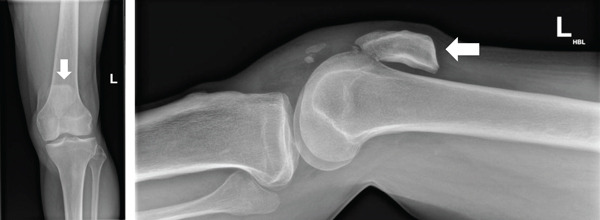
Left knee radiograph demonstrating superior displacement of the patella (arrow), consistent with patella alta and disruption of the extensor mechanism, in keeping with patellar tendon rupture.
